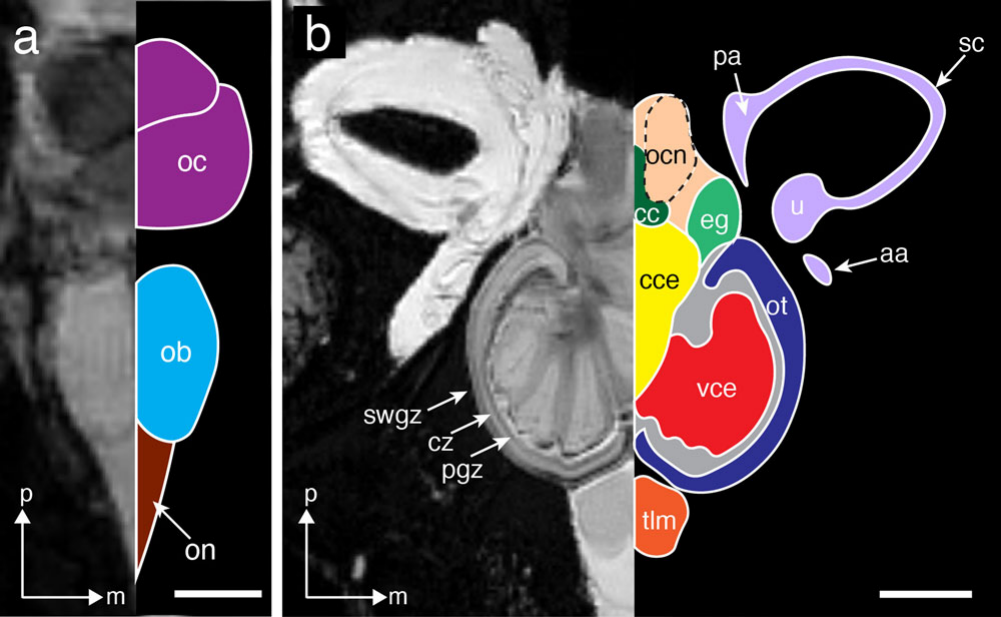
(a) Transverse MRI 2D slice through the olfactory bulb. The crossing of the optic nerves at the chiasm is also visible. (b) Mid‐transverse MRI 2D slice. The MRI image is on the left, and line tracings on the right indicate different regions. Each region is colored with colors corresponding to the 3D segmentations, while the ventricle and areas that cannot be differentiated are rendered in gray. Inner ear structures are colored with light purple. Dashed line indicates the likely boundary. The membranous structures of the inner ear are also shown adjacent to the brain. Orientation: m, medial; p, posterior. aa, anterior ampulla; cc, cristae cerebelli; cce, corpus cerebelli; cz, central zone of the ot; eg, eminentia granularis; ocn, octavolateral nucleus; ot, optic tectum; pa, posterior ampulla; pgz, periventricular gray zone of the ot; sc, semicircular canal; swgz, superficial gray and white zone of the ot; tlm, telencephalon medial lobe; u, utricle; vce, valvula of cerebelli. Scale bars, 2.5 mm (a); 5 mm (b).
